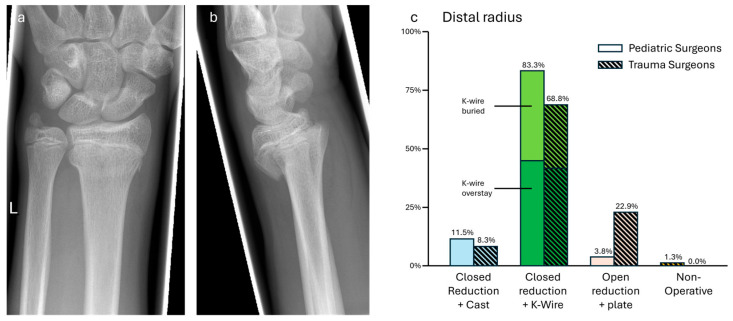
(a) Anterior–posterior and (b) lateral X-rays of a distal radial fracture with concomitant non-dislocated fracture of the styloid process of the distal ulna (m, 17 y, 185 cm, 85 kg). (c) Descriptive data of the survey results, indicated in percentages.
